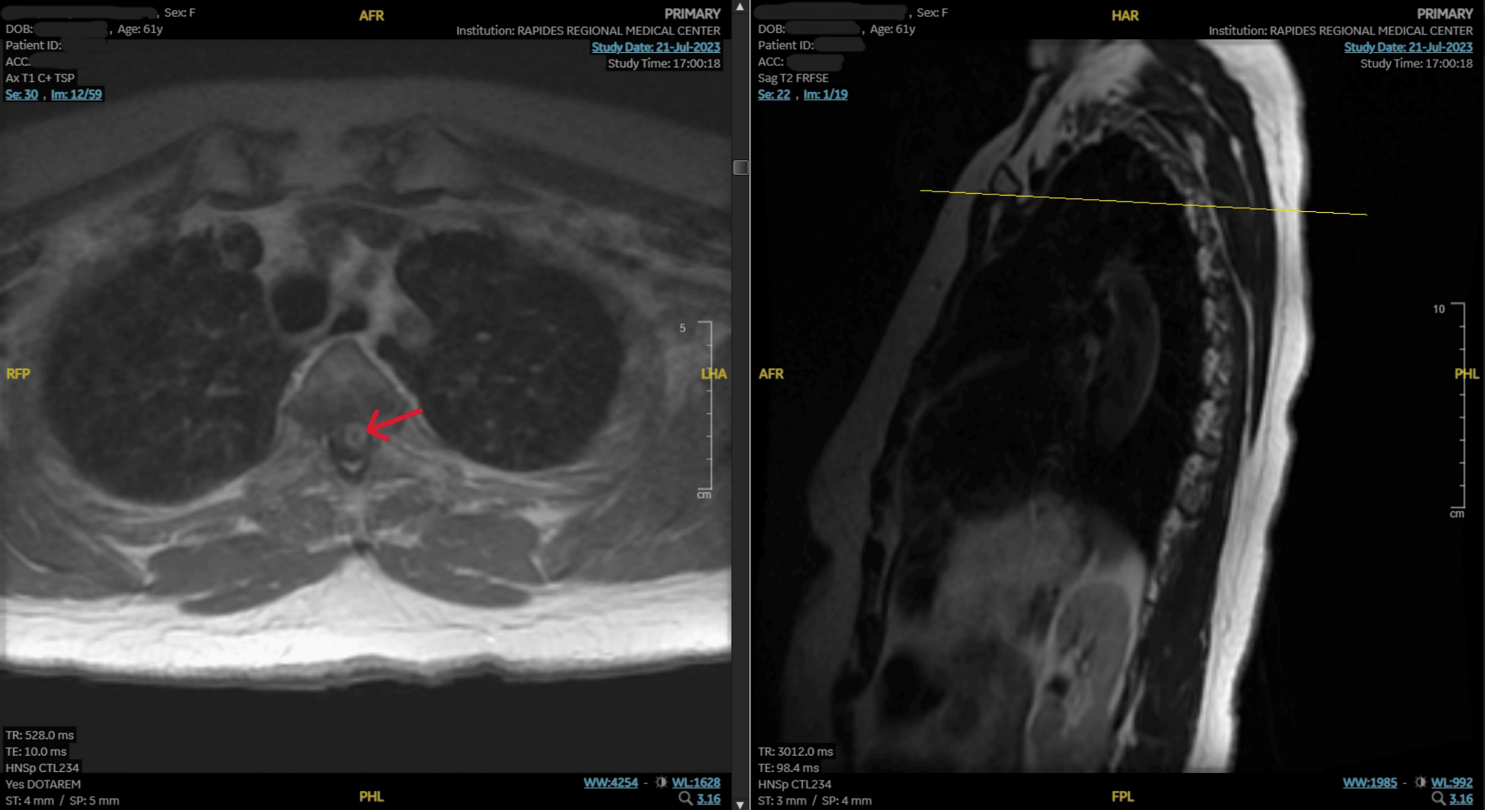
MRI demonstrating a new enhancing lesion in the upper thoracic cord MRI, magnetic resonance imaging.
